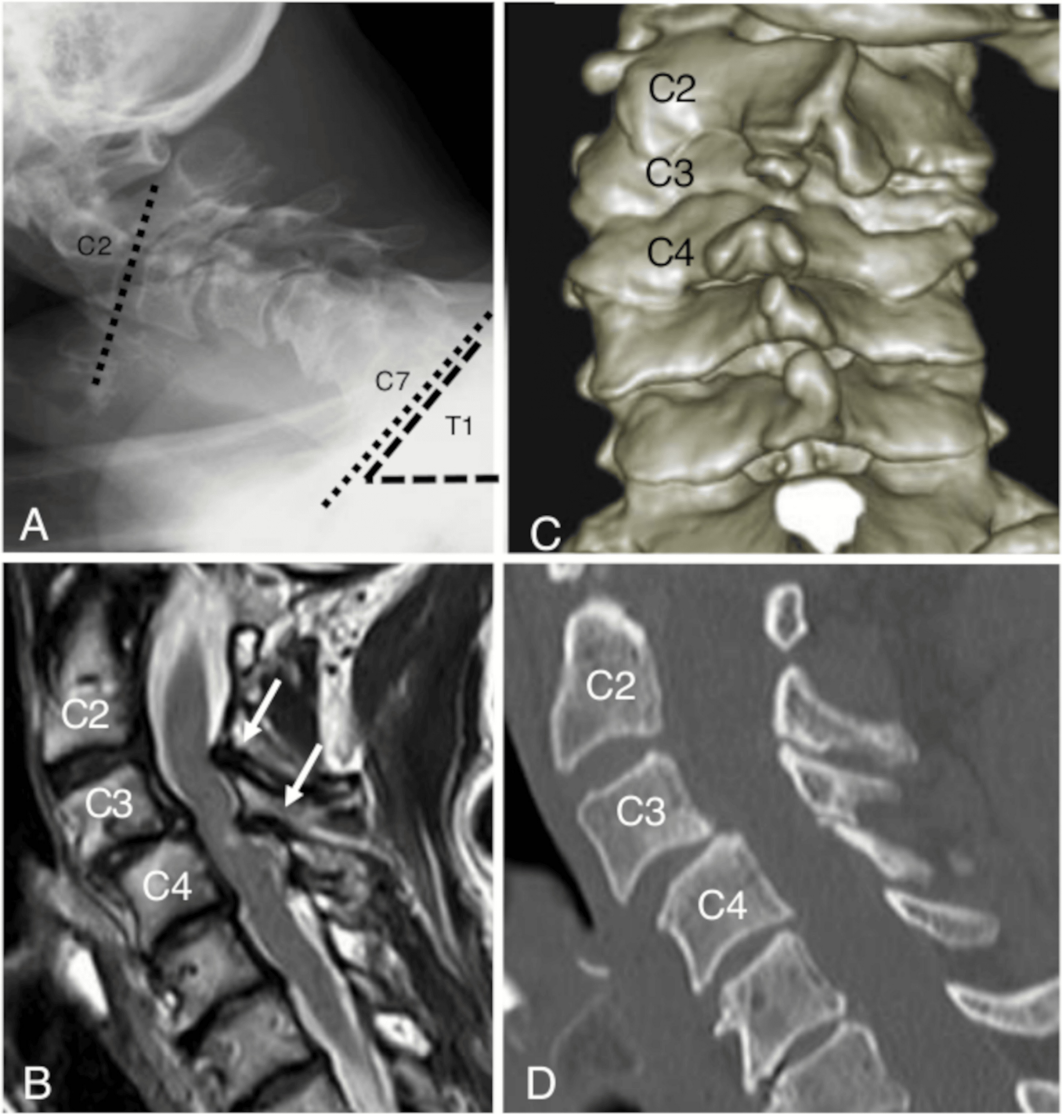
Preoperative images (A) On X-ray, the C2-C7 Cobb angle (dotted lines) and T1 slope (dashed lines) were -13° and 55°, respectively. (B) A sagittal magnetic resonance image revealed severe cervical canal stenosis from C2 to C4 (white arrows). The canal stenosis resulted in myelopathy at the same levels. (C, D) Anterior spondylolisthesis at C3/C4 and scoliotic change were concurrently diagnosed on plain and 3D reconstructed computed tomography images.
